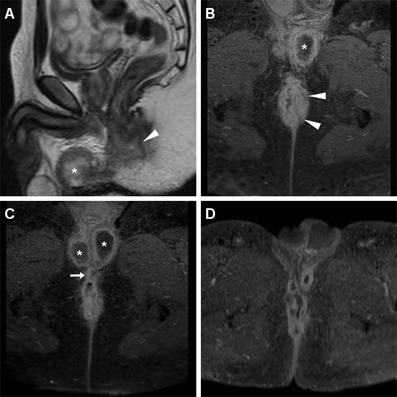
A 39-year-old HIV-infected man with biopsy-proven SCAC. Staging MRI confirms left-sided anal thickening (*arrowheads*) with abnormal T2 signal intensity (a) and strong contrast enhancement (b). Incidentally, two abscess collections with necrotic content and peripheral enhancement are seen ventrally, connected to the anal canal by a fistulous track (*arrow* in c). Subtotal regression of changes after treatment is seen on follow-up MRI (d)
